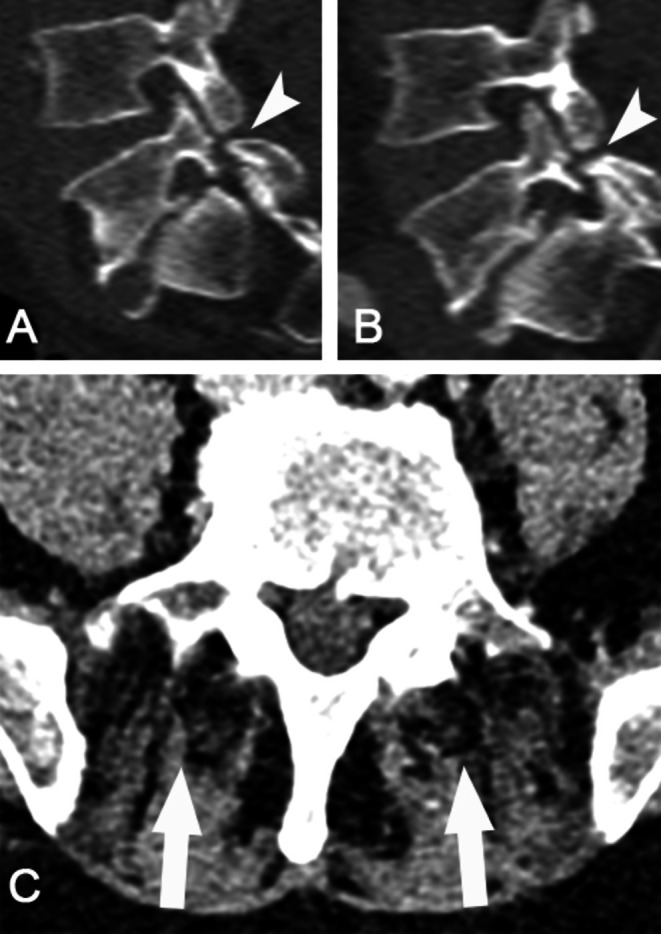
Sagittal (A‐B) and axial CT images (C) of L5‐S1 IS. The bilateral isthmic lysis presents anterolisthesis of the L5 vertebral body over S1 (arrowheads in A‐B). The axial image (C) shows diffuse moderate atrophy and FI of the paravertebral muscles, particularly of the MF (arrows in C).
